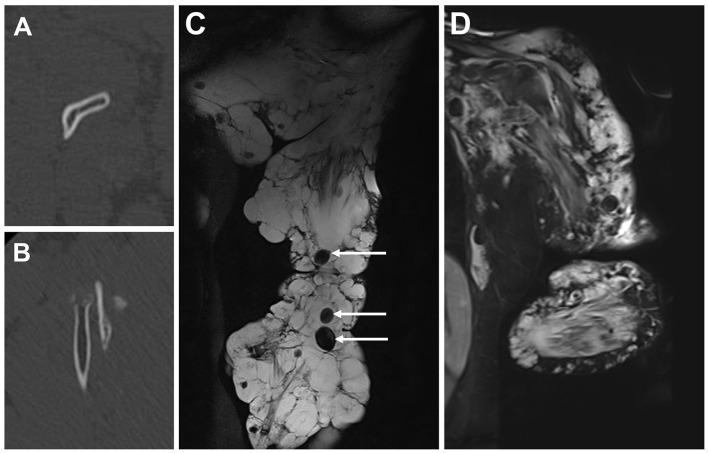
Radiology findings on admission. Computed tomography with bone window settings demonstrated radiolucency in the medulla of the left humerus (A) and radius (B). T2-weighted magnetic resonance imaging (C) revealed a multicystic, marked hyperintensity infiltrating the left upper extremity. Phleboliths presented as signal voids (arrows). Magnetic resonance imaging at 9-month follow-up (D) showed a decrease in signal intensity compared to (C).
